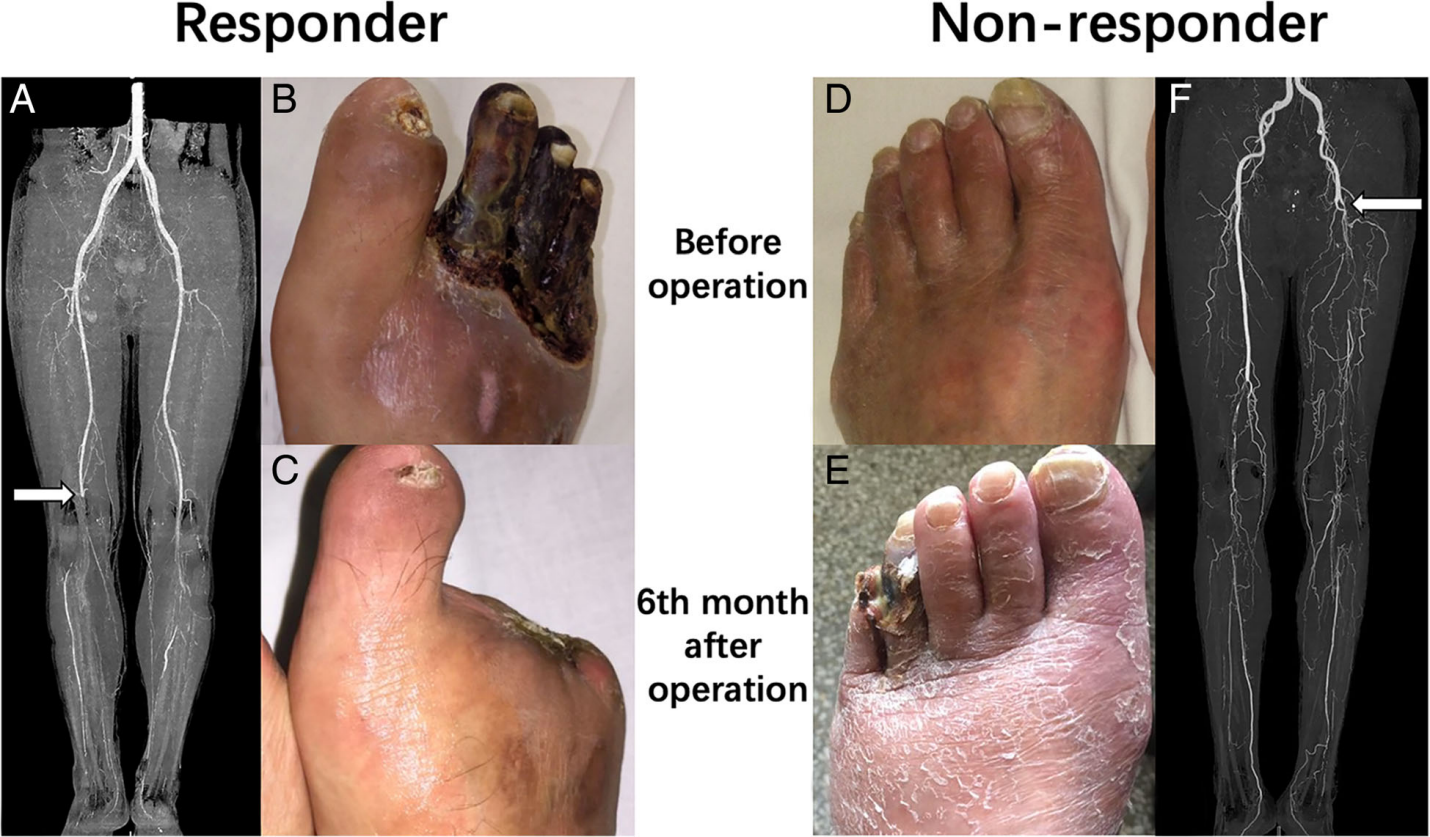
Typical preoperative computed tomographic angiography (CTA) and longitudinal foot photos of a responder (a, b, c) and a non-responder (d, e, f). The case on the left side was a 37-year-old male diagnosed with thromboangiitis obliterans, characterized by progressive limb gangrene (b), occlusion level at popliteal artery (a), transcutaneous oxygen pressure of 15 mmHg, Log transplanted CD34+ cells of 8.17, and baseline blood fibrinogen < 4 g/L. The nomogram-based score was 192, indicating a probability of 0.76 to be a responder. His toe gangrene was debrided during the transplantation, and the wound healed completely within 6 months (c). The case on the right side was a 73-year-old male diagnosed with atherosclerosis obliterans, characterized by left foot rest pain, occlusion level at proximal superficial femoral artery (f), transcutaneous oxygen pressure of 12 mmHg, Log transplanted CD34+ cells of 7.38, and baseline blood fibrinogen < 4 g/L. The nomogram-based score was 110, indicating a probability of 0.18 to be a responder. His rest pain was not relieved and the fourth and fifth toes had gangrene at 6 months (e). The white arrows indicate the arterial occlusion level
